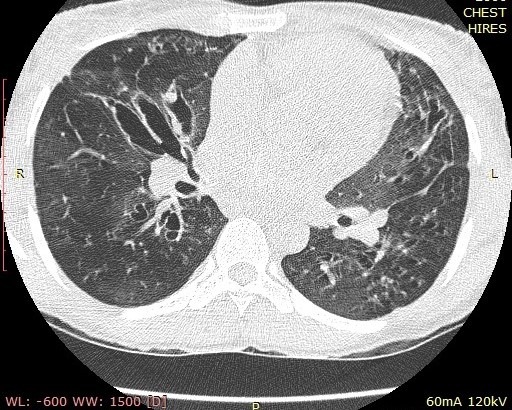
Chest Axial CT scan showing cystic and cylindrical dilatation of the bronchi with bronchial wall thickening centrally, more severe on the left
